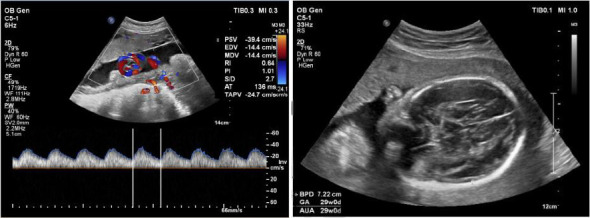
Fetal ultrasound: late intrauterine pregnancy, breech presentation (case 9).
